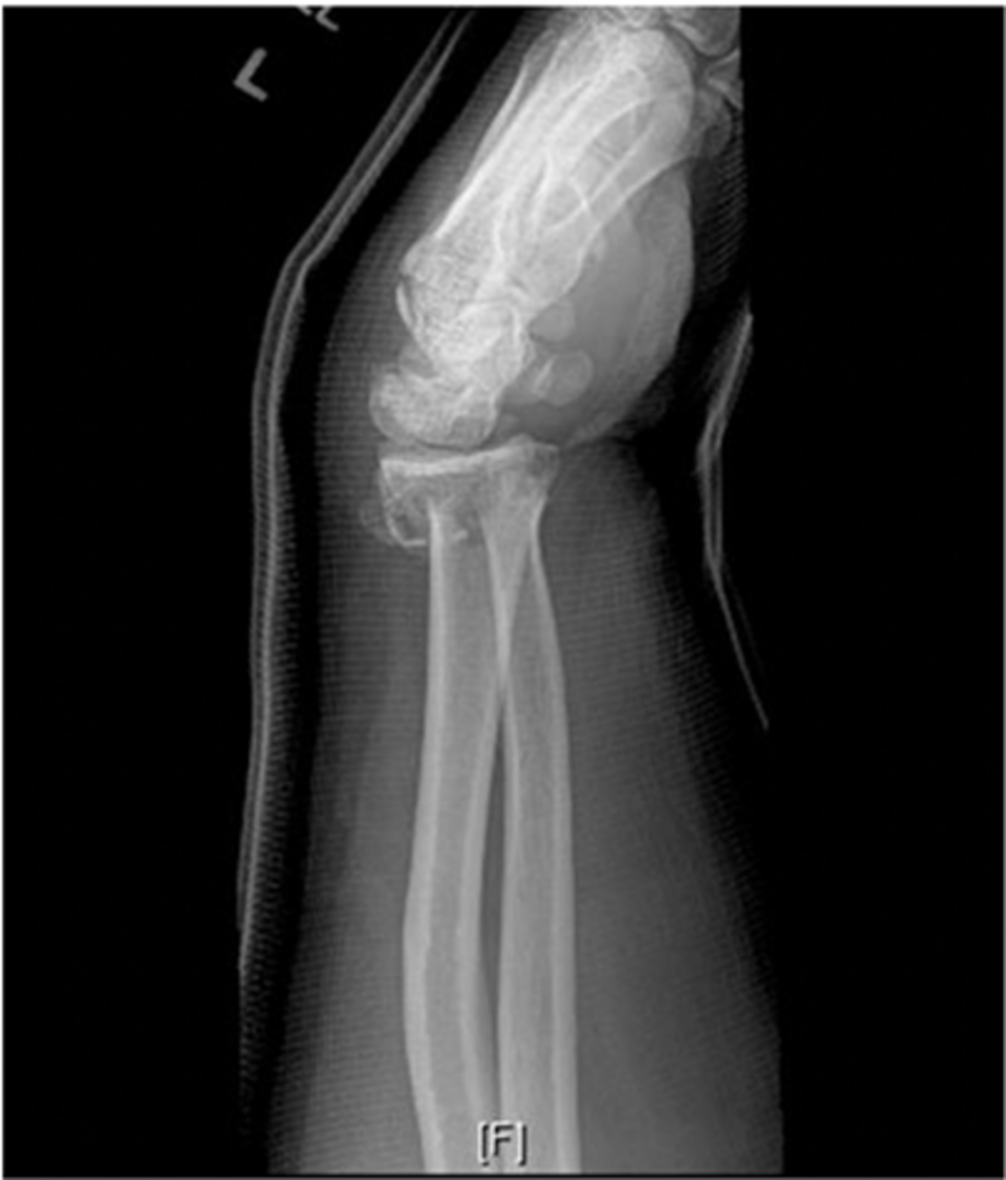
Lateral X-ray of the left wrist showing comminuted distal radius fracture with dorsal angulation of the distal fragment and a displaced ulnar styloid fracture
